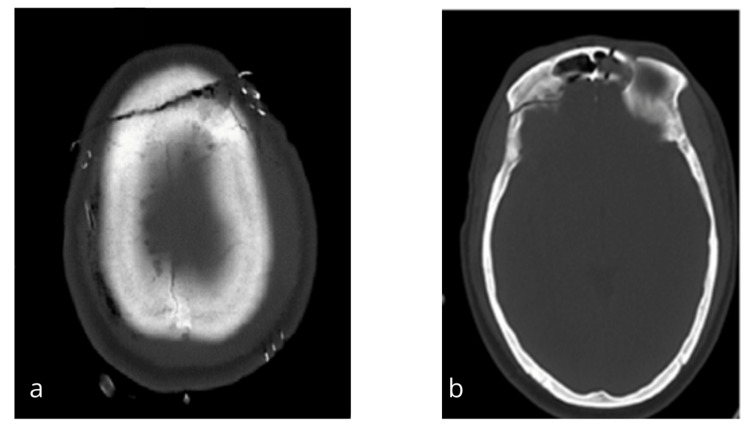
(a) Preoperative CT head (bone window) showing segmented frontal bone. (b) Preoperative CT head showing the fracture line extending to the frontal sinus.
